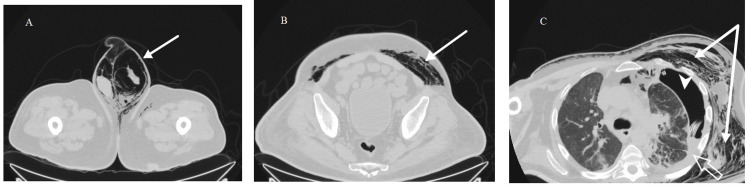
CT of the chest, abdomen, and pelvis showing the pneumoscrotum, the subcutaneous emphysema, and the pneumothorax. (A) CT showing bilateral pneumoscrotum (arrow). (B) CT showing a subcutaneous emphysema mostly localized in the left abdominal wall (arrow). (C) CT showing a subcutaneous emphysema in the left thoracic wall (arrow), left-sided pneumothorax (arrow head), and left-sided pleural effusion (empty arrow).
